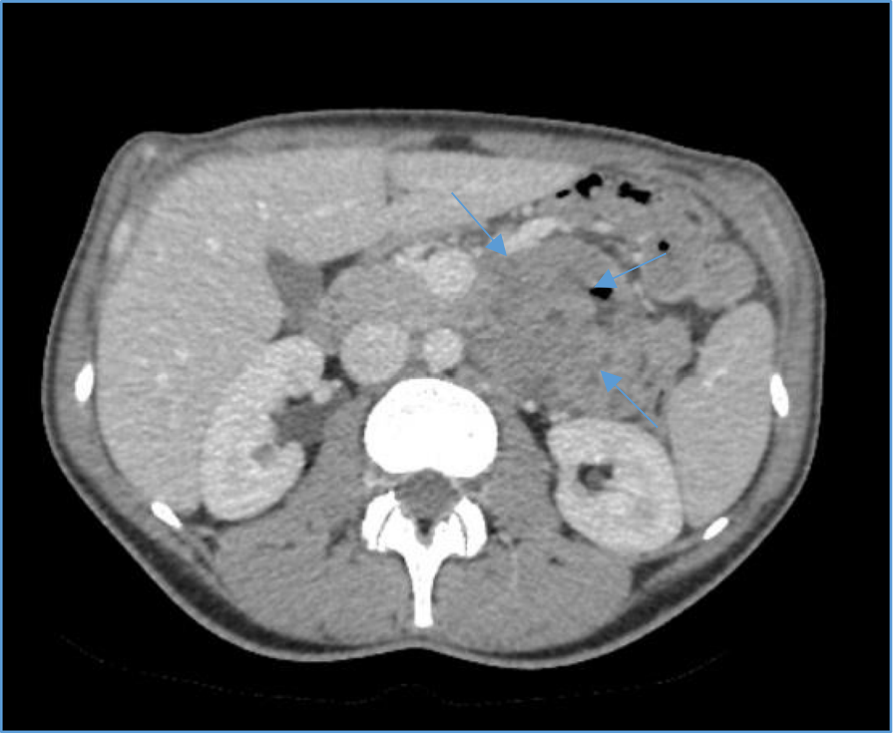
Computed tomography scan of the abdomen. Axial transverse view of 4.7 × 6.2 × 6.5 cm non-homogenic tumour (blue arrows) with a cystic component contacting with an inferior mesenteric vein
